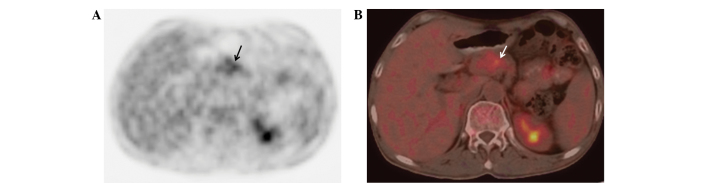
Positron emission tomography-computed tomography (PET-CT) manifestations of glucagonoma syndrome. (A and B) PET-CT revealing the presence of a lesion with mild ^18^F-fludeoxyglucose uptake (arrow), which corresponds to the location of the tumour identified by CT and magnetic resonance imaging.
